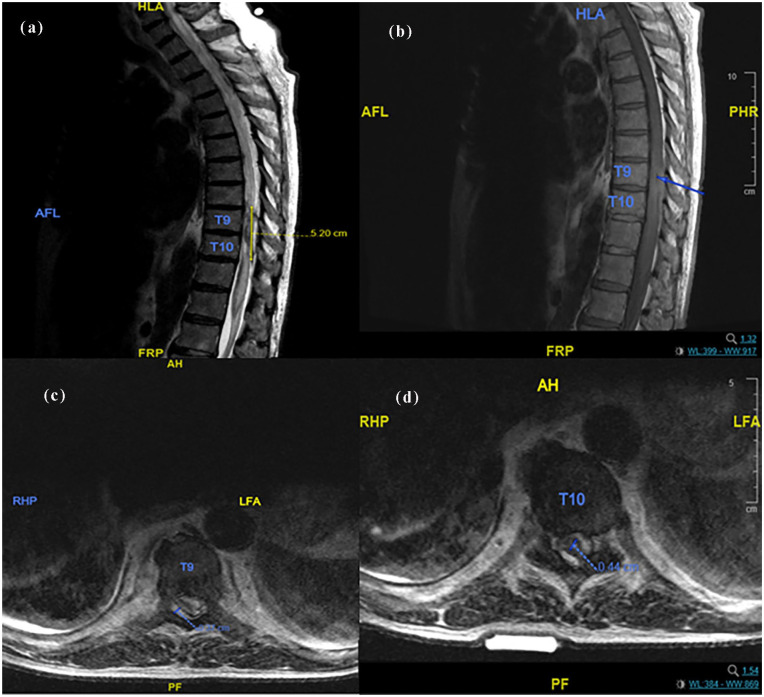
Thoracic MRI without contrast. (a) Sagittal. T2-weighted, demonstrating diffuse hyperintensity, suggestive of cord edema. (b) Sagittal. T1-weighted, indicating tip of the intrathecal catheter at T9 (blue arrow). (c) Axial. T2-weighted, showing right dorsal epidural fluid collection, measuring 0.37 cm, with mass effect. (d) Axial. T2-weighted at T10 level demonstrating heterogeneous collection suggestive of granuloma or hematoma measuring 0.44 cm.
